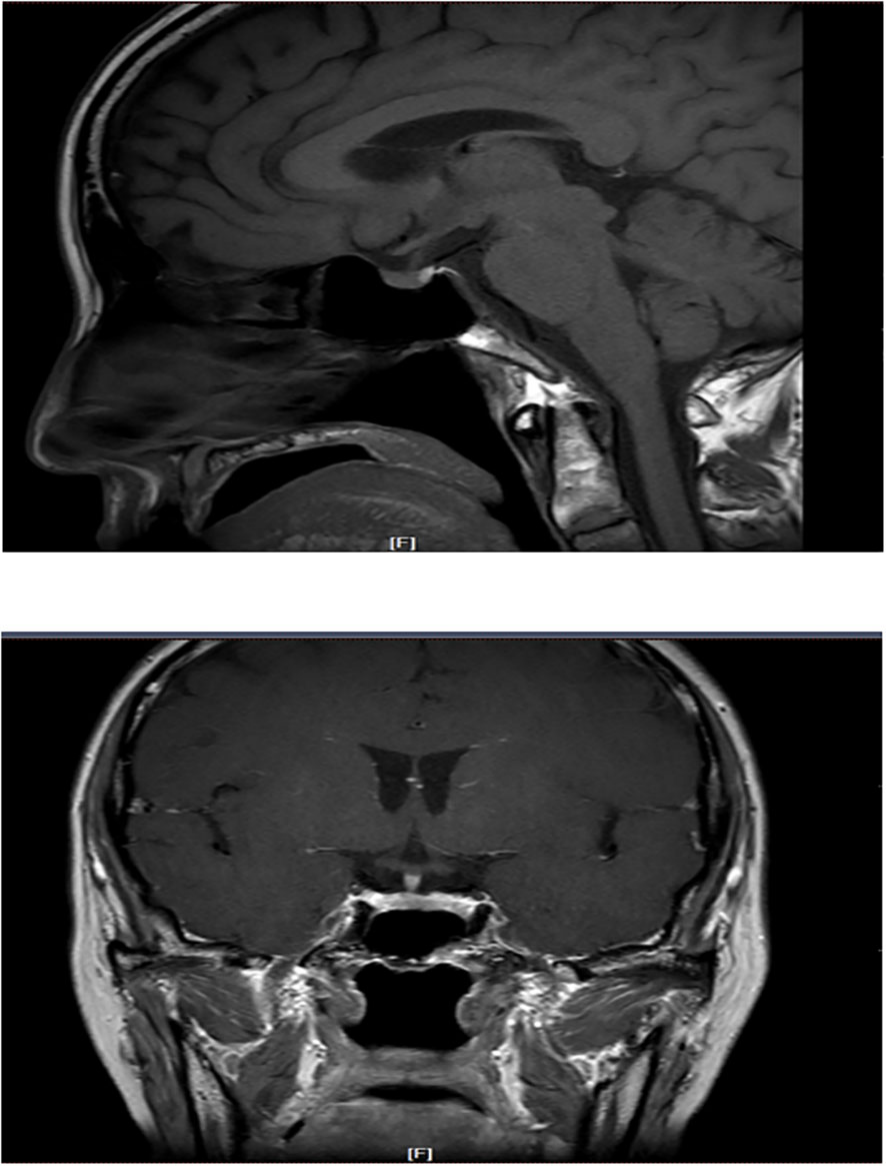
MRI with contrast showing a normal-sized pituitary and normal enhancement.
